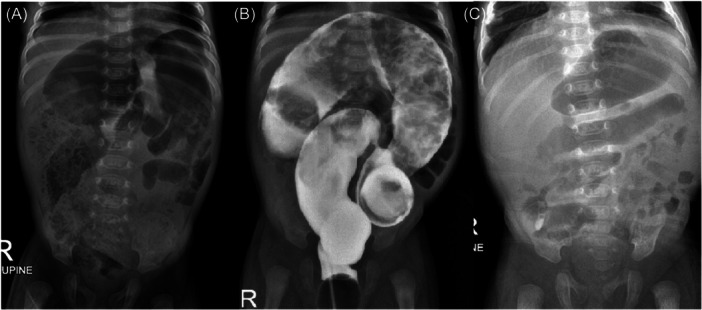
A portable anteroposterior supine abdominal radiograph of the 6‐week‐old infant (A) revealed diffuse colonic dilatation with moderate amount of fecal material in colon, more pronounce at right‐side colon (allows); (B) after bedside barium enema, showed a focal severe luminal narrowing (arrow) of the proximal sigmoid colon proximal colonic dilatation and retained fecal content. (C) after conservative treatment, revealed interval decreasing degree of the colonic dilatation as well as a much‐decreasing amount of the retained fecal material. Noted no abnormal small bowel dilatation. R, right side.
